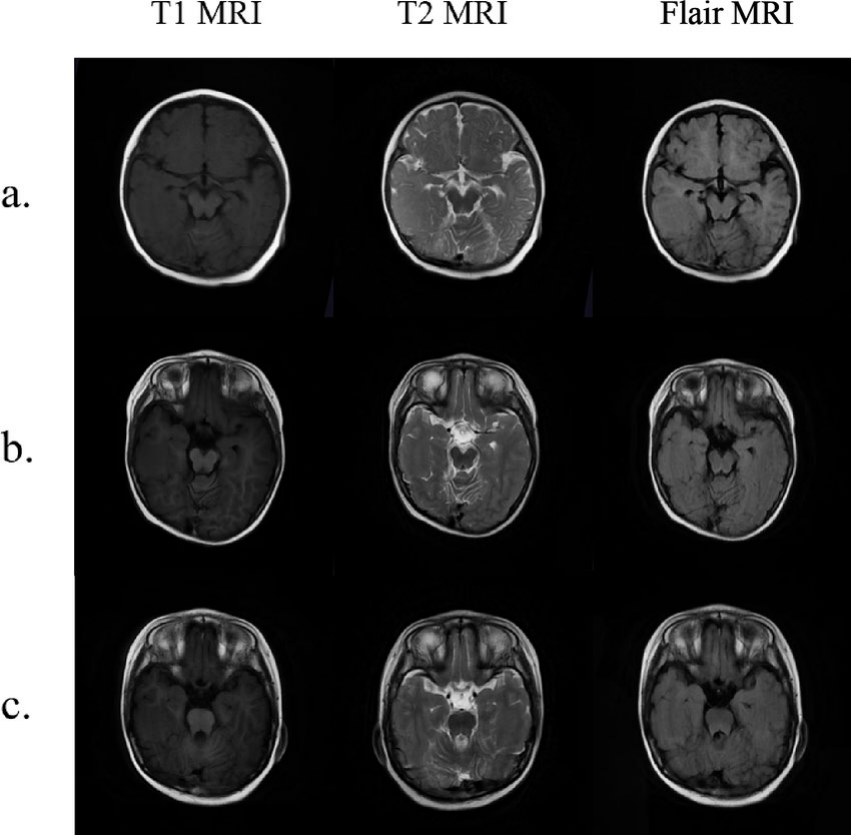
Axial MRI demonstrates widening of the right temporal lobe gyrus. (a) 7 months of age; (b) 2 years and 9 months of age; (c) 4 years and 8 months of age.
